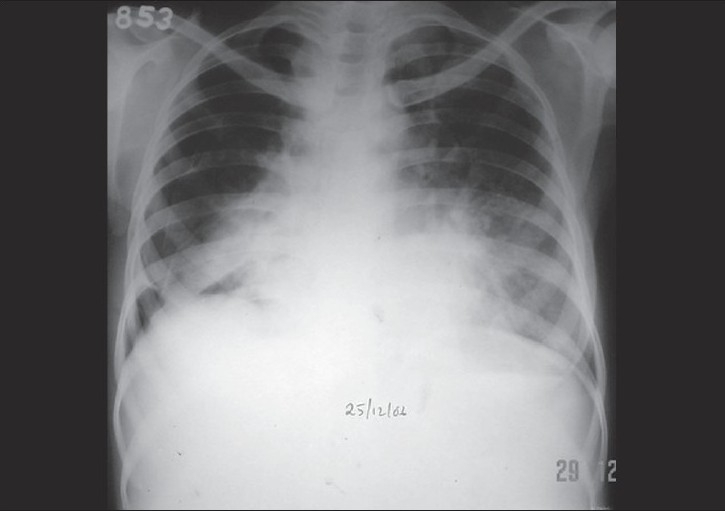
A chest radiograph showing right-sided pneumothorax
